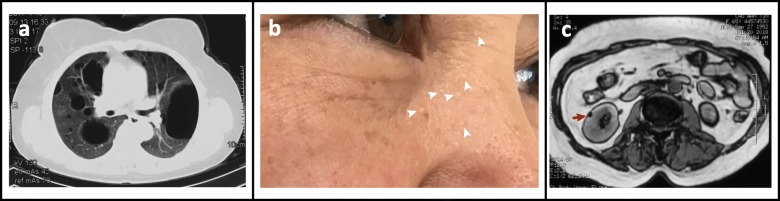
Representative manifestations in lung, skin and kidney observed in Chinese BHDS patients. a Chest CT showing multiple lung cysts in Patient 10. b Typical skin lesions (withe arrow heads) observed in Patient 7. c Abdominal MRI showing a renal cyst in patient 31 indicated by the red arrow
